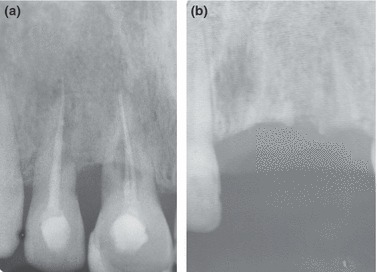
(a) Radiographic examination of the 11 and 12 showed external and internal inflammatory resorption and external replacement resorption. (b) The 11 and 12 were accessed and gutta percha removed and were subsequently decoronated with surgical closure.
